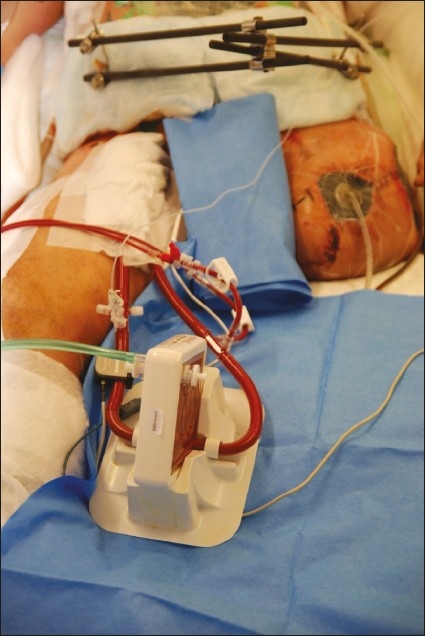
PECLA system with gas exchange membrane visible, along with ultrasound chip for measurement of blood flow.
